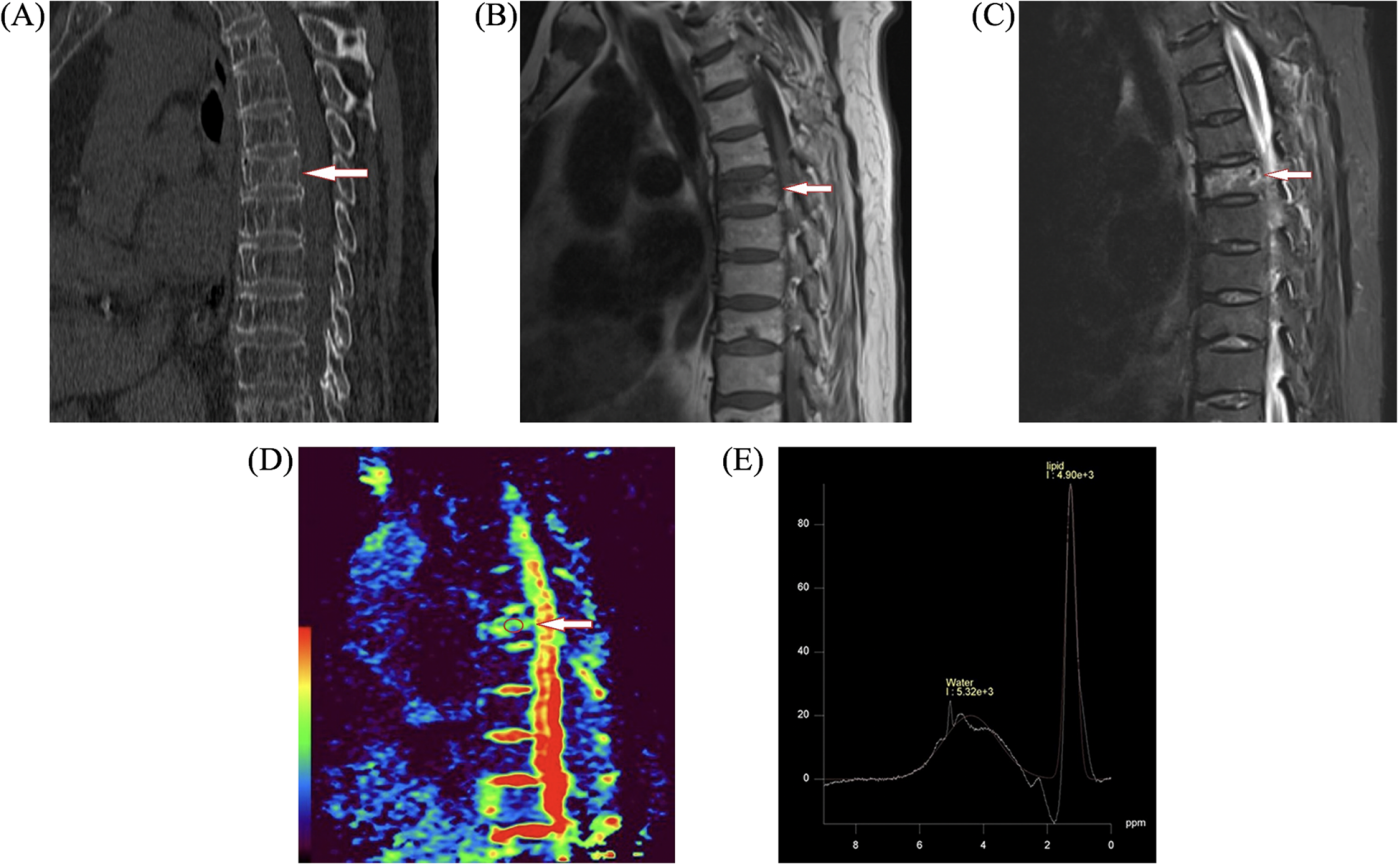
A 67-year-old woman with osteoporotic fracture of T7 vertebra. a Mid sagittal CT shows vertebral compression changes and osteoporotic changes (arrow). b, c T1-weighted and T2-weighted images. The lesion is hypointense on T1-weighted image (arrow) and hyperintense on T2-weighted image (arrow). d IVIM-DWI image, high signal, and regions of interest were placed within lesion (circle), *f* = 0.128, *D* = 1.65 × 10^−3^ mm^2^/s, *D = 1.84 × 10^−2^ mm^2^/s. e MRS, LPA = 4900, WPA = 5320, lipid fraction of 47.79 at T7
